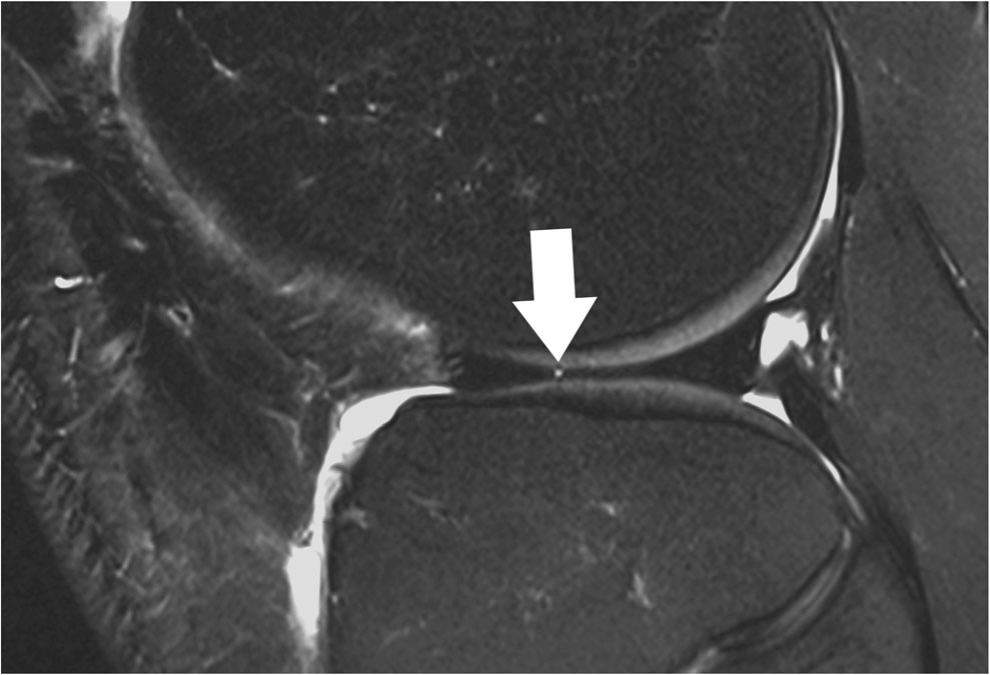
Sagittal T2-weighted, fat-suppressed fast spin echo image of the right knee in a 39-year-old male patient acquired at 3 Tesla (Siemens Healthcare, Erlangen, Germany) using a dedicated 28 channel transmit-receive knee coil shows a small radial tear of the lateral meniscus (*arrow*)
